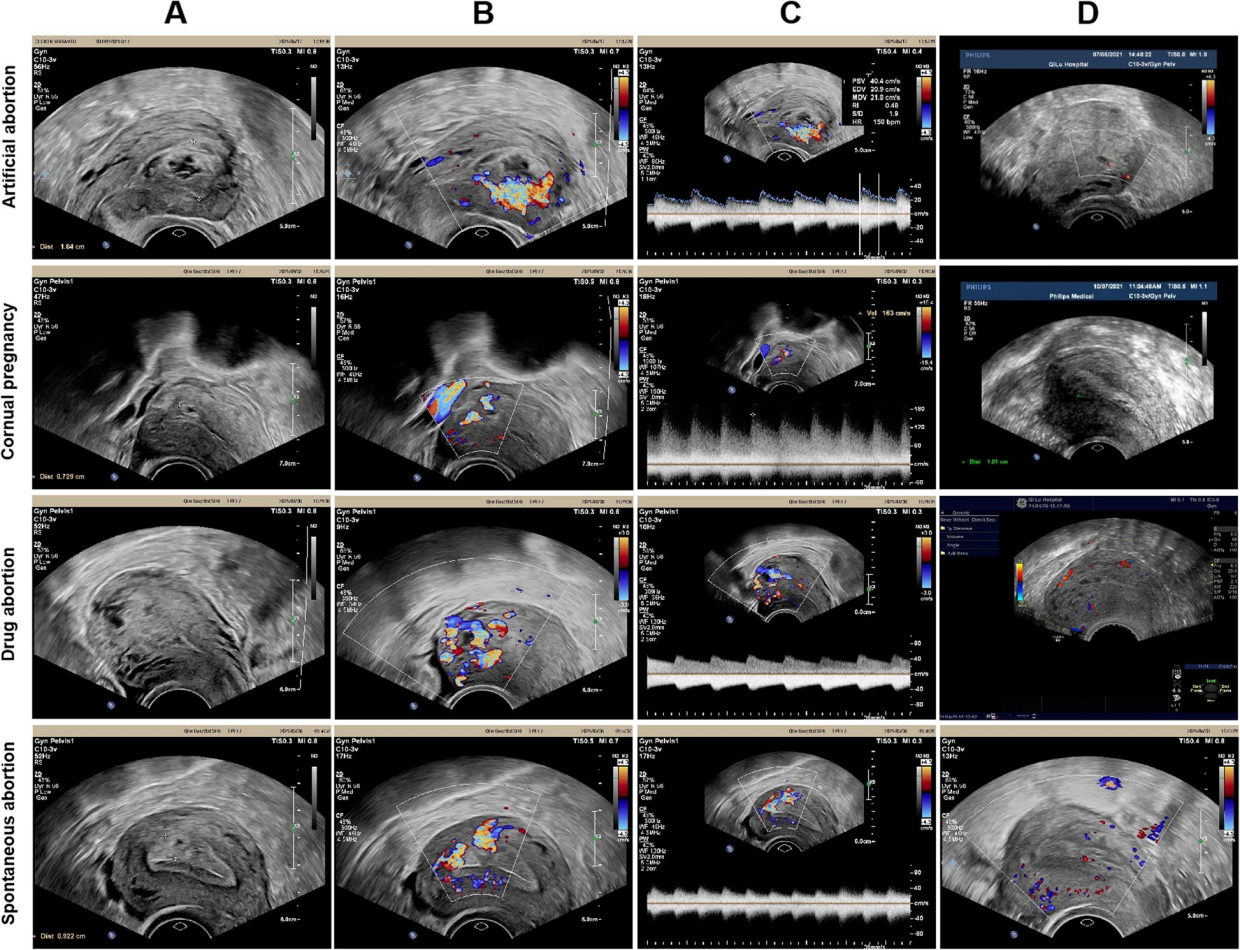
Ultrasound images of patients with pregnancy-related UAVMs. This figure show four patients with pregnancy-related UAVMs. Their related disease are artificial abortion, cornual pregnancy, drug abortion and spontaneous abortion. A show the uterine cavity and the UAVM lesion. B show the blood flow of the UAVM lesion. C show the spectrogram of the UAVM lesion. D show the situation after surgery
